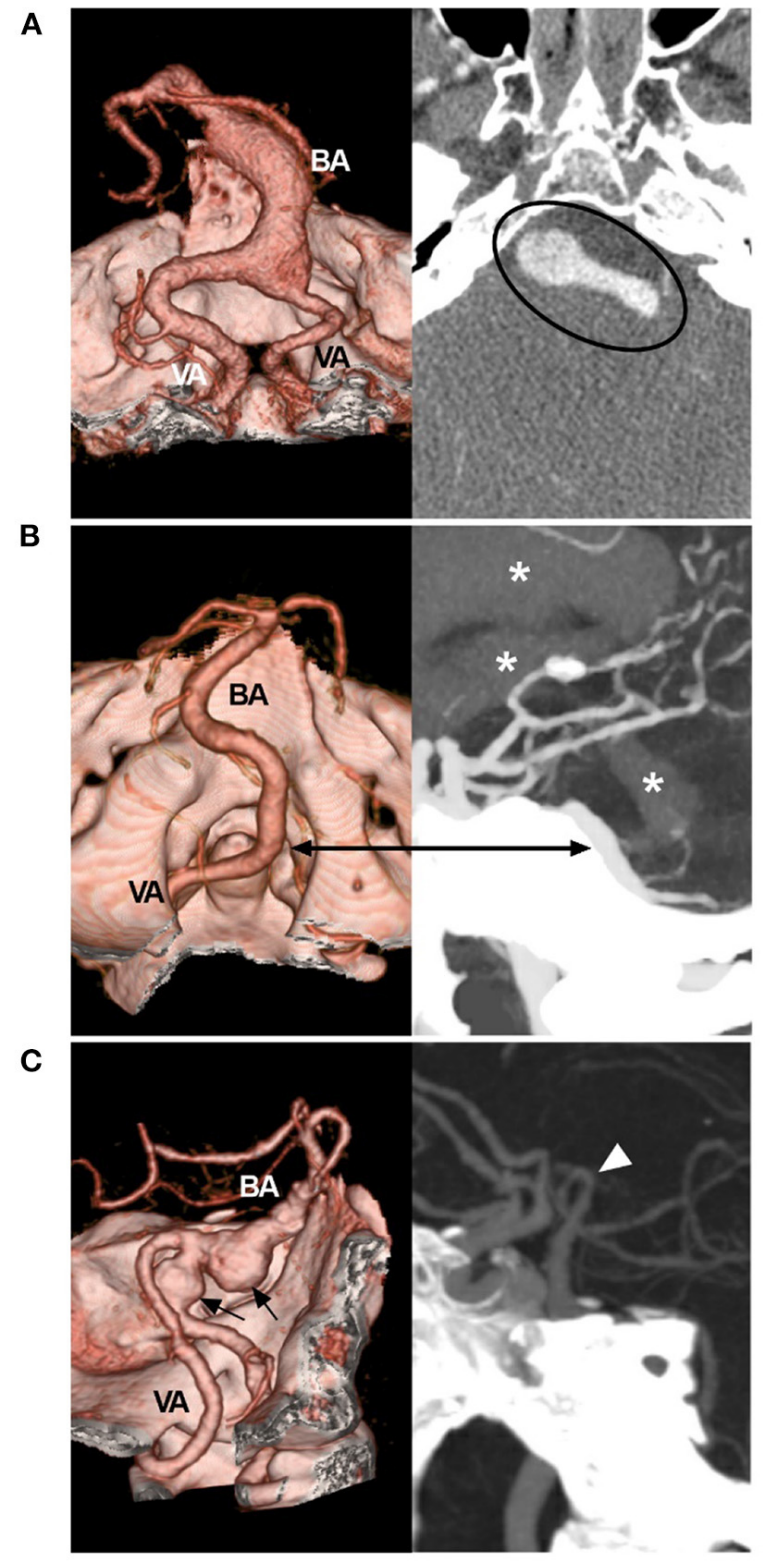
Symptomatic VBD on CTA. (A) Left: CTA reconstruction image showing a dilated VBD with mass effect; right: MIP image showing the VBD compressing the brainstem (circle). (B) CTA reconstruction image (left) and MIP image (right) of the rupture point of the VBD (bidirectional arrow); asterisks indicate hemorrhage extension from the fourth ventricle to the third and lateral ventricles. (C) Left: CTA reconstruction image showing VBD with two aneurysms (arrows); right: MIP image showing the BA bifurcation (arrowhead) reaching the floor of the third ventricle. BA, basilar artery; CTA, computed tomography angiography; MIP, maximum intensity projection; VA, vertebral artery; VBD, vertebrobasilar dolichoectasia.
